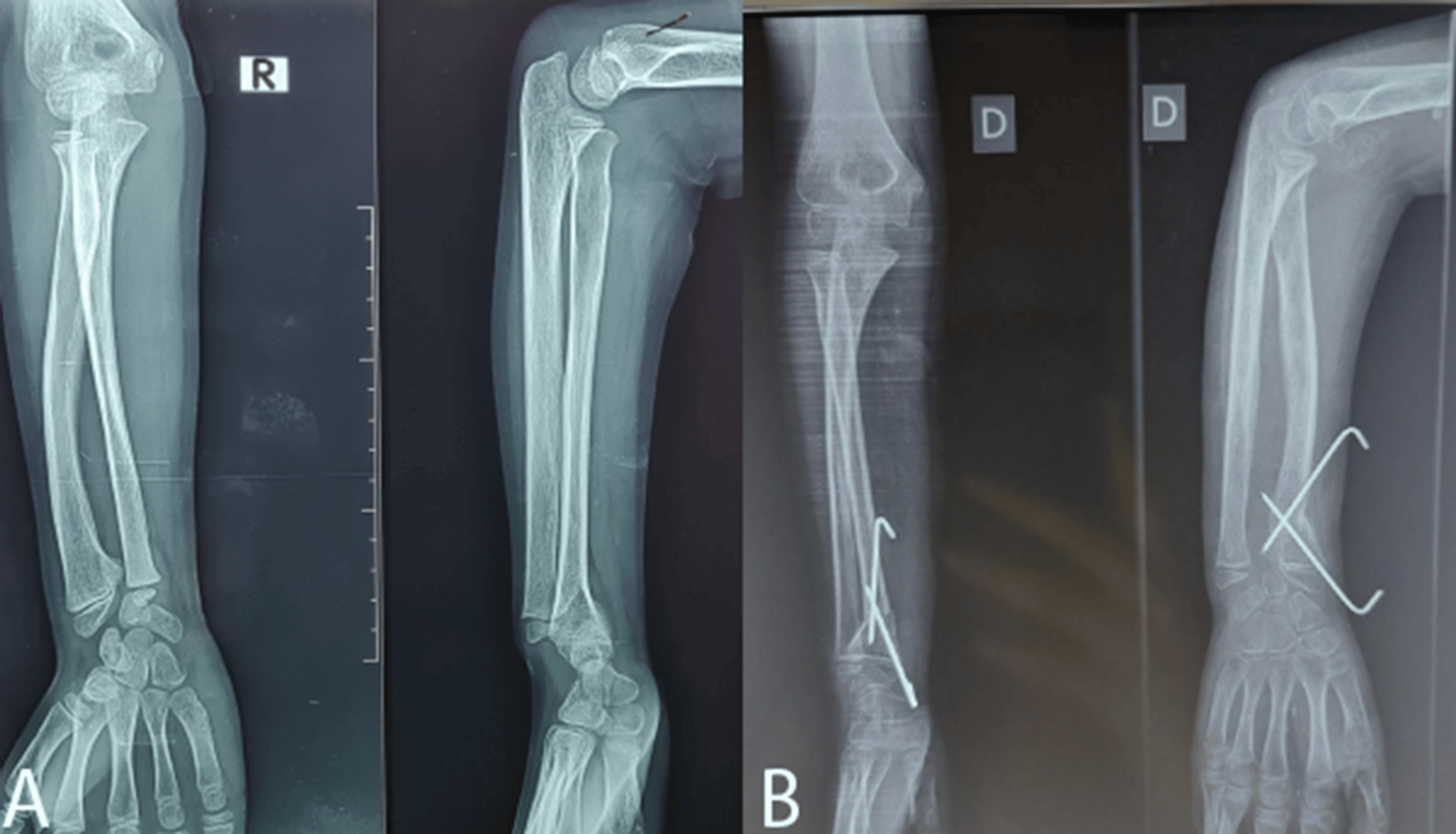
Wrist radiographs in the sibling with Leri-Weill syndrome: (A) preoperative radiographs and (B) postoperative radiographs
